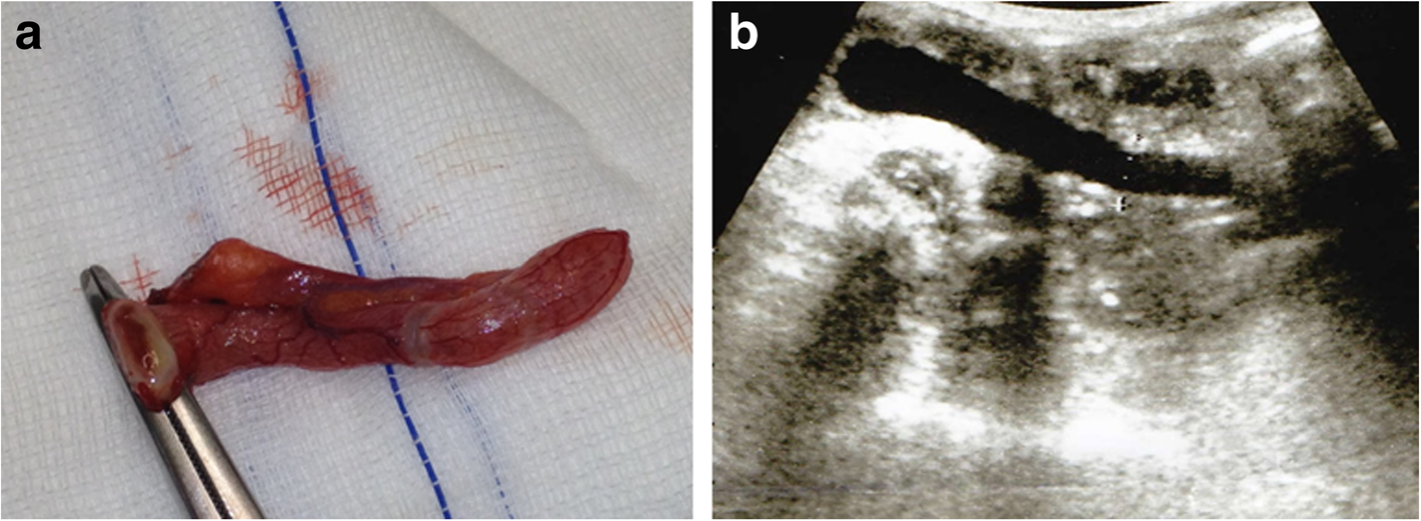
Acute catarrhal appendicitis (a); abdominal ultrasound showing “finger in glove” anechoic image with incompressible lumen (b).
